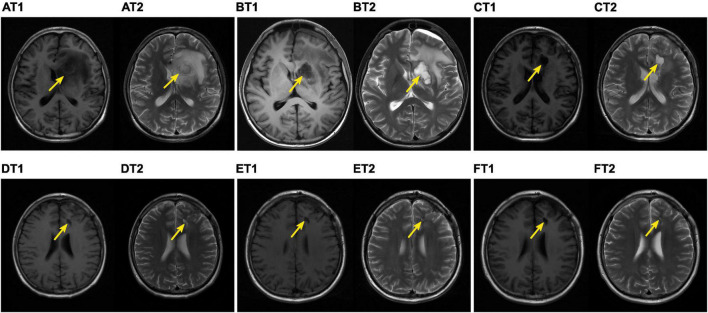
Brain magnetic resonance imaging (MRI) findings. T1, magnetic resonance T1-weighted imaging. T2, magnetic resonance T2-weighted imaging. On day + 138 after haematopoietic stem cell transplantation (HSCT), the patient experienced persistent headache and violent mood swings. The follow-up MRI-T1 (A_*T*1_) and MRI-T2 (A_*T*2_) identified signal abnormalities (see yellow arrows) suggestive of left-side basal ganglia abscesses (28 × 18 mm) and signs of perifocal oedema (pre-operation). On day + 141 after HSCT, intracranial debridement was performed on the child. On day + 164 after HSCT, MRI-T1 (B_*T*1_) and MRI-T2 (B_*T*2_) showed that the basal ganglia mucormycosis was completely removed post-operation. On day + 183 after HSCT, MRI-T1 (C_*T*1_) and MRI-T2 (C_*T*2_) were performed post-operation. On day + 216 after HSCT, MRI-T1 (D_*T*1_) and MRI-T2 (D_*T*2_) were performed post-operation. On day + 251 after HSCT, MRI-T1 (E_*T*1_) and MRI-T2 (E_*T*2_) were performed post-operation. On day + 339 after HSCT, MRI-T1 (F_*T*1_) and MRI-T2 (F_*T*2_) revealed that the surgical site appeared to be healing well with a gradual reduction in size.
